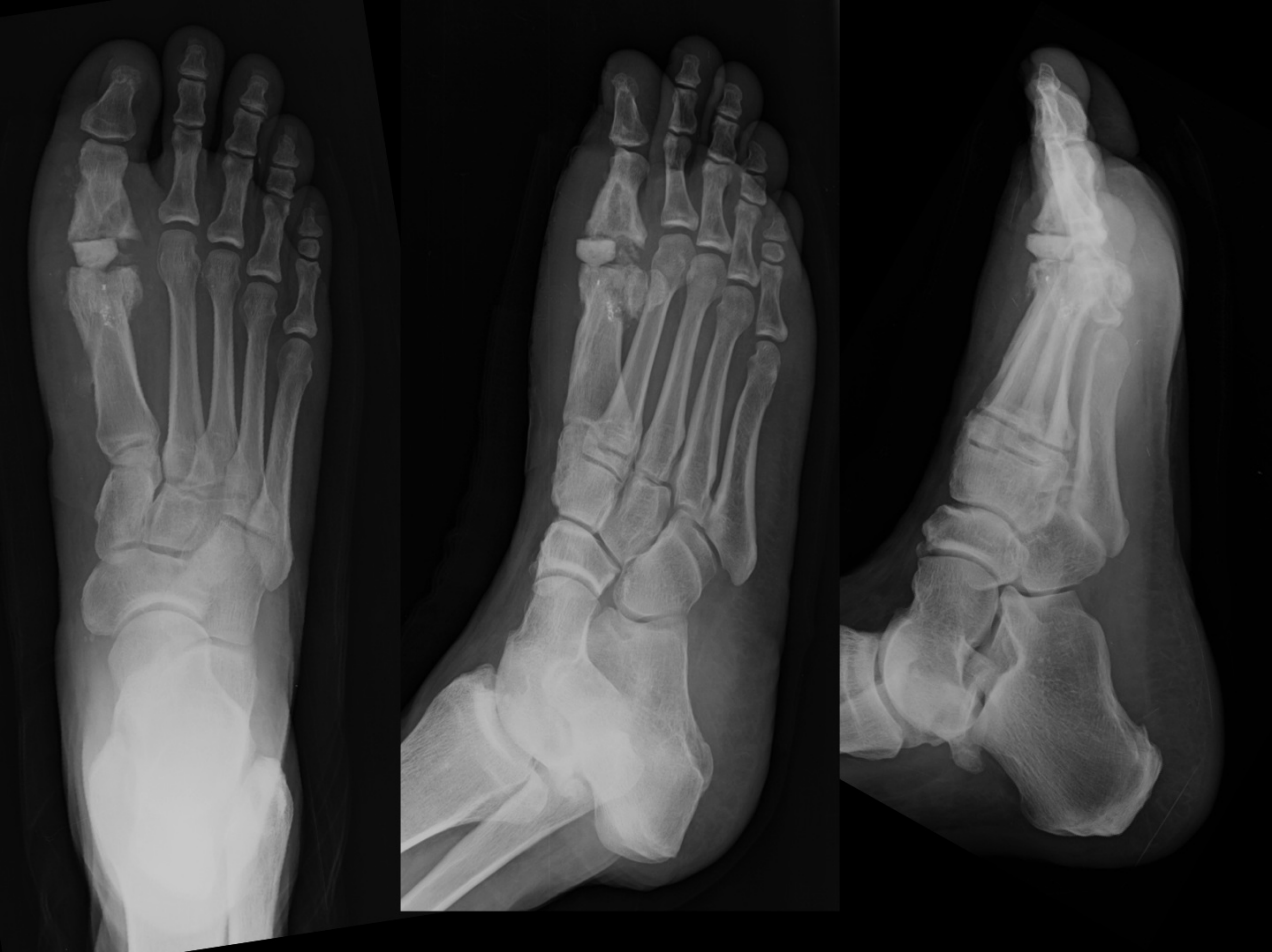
Postoperative radiographs Postoperative AP, oblique, and lateral foot radiograph demonstrating the placement of the articulating antibiotic spacer within the first MTP joint.
